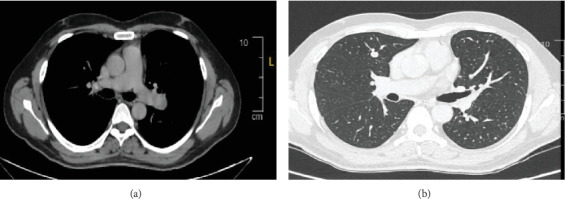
(a) Contrast-enhanced CT chest showing a left hilar lymph node measuring 2.5 × 2.2 *cm*. (b) Multiple pulmonary nodules, the largest of which is located in the right middle lobe, measuring approximately 9 mm.
